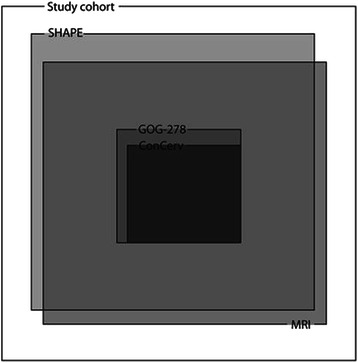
Distribution of less radical surgery candidates according to the various criteria. The area of square with gray per white is proportional to the number of less radical surgery candidates per study cohort. Study cohort, 100% (n = 125); ConCerv, 8.8% (n = 11); GOG-278, 11.2% (n = 14); SHAPE, 62.4% (n = 78); MRI, 59.2% (n = 74).
